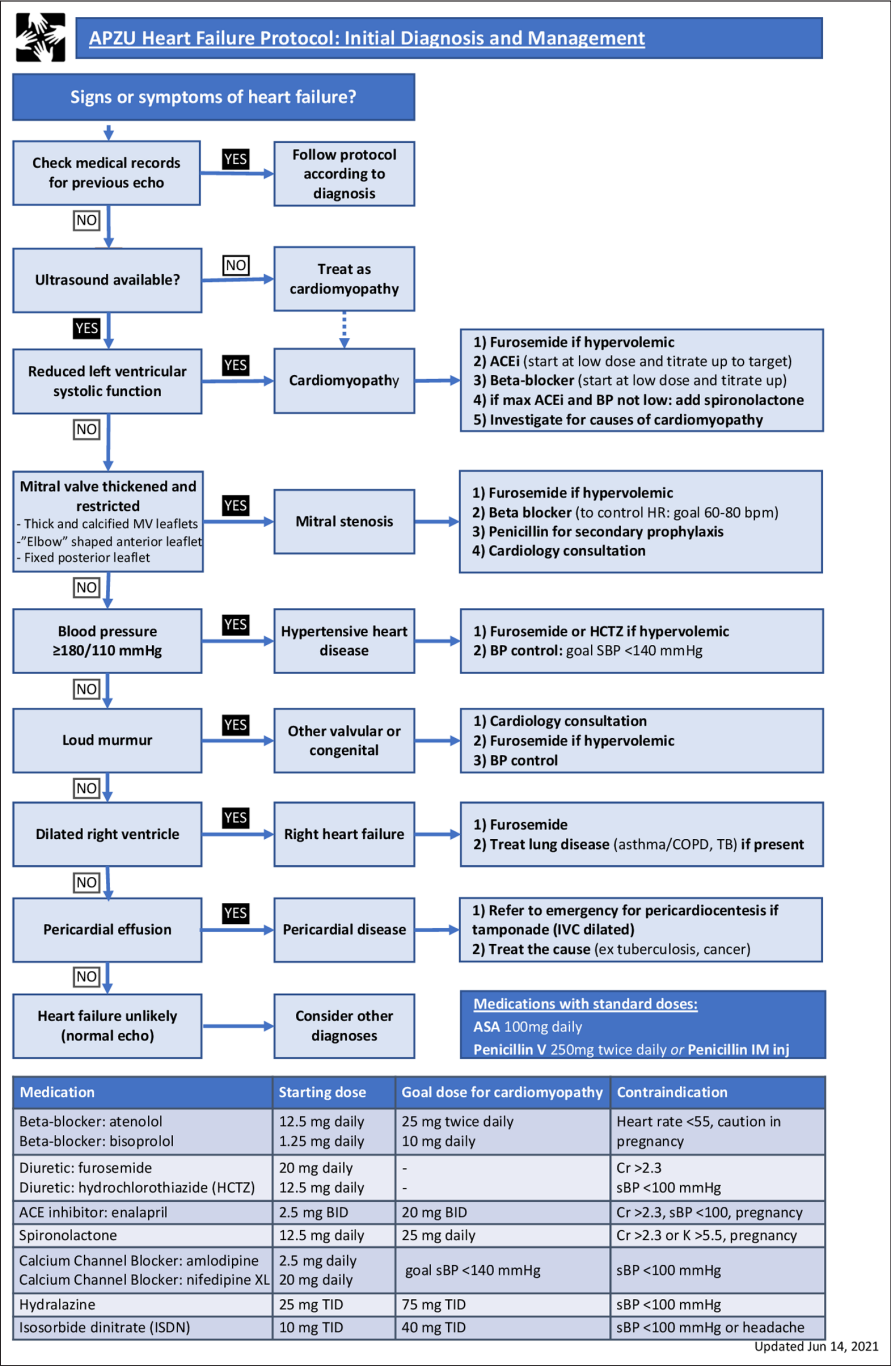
Heart failure protocol for initial diagnosis and management used by non-physician providers in PEN-Plus clinic in Neno, Malawi. Providers use focused cardiac ultrasound and follow the flow on the left-hand side of the protocol to determine a diagnostic category and management. The right-sided boxes guide management based on the diagnosis made.
